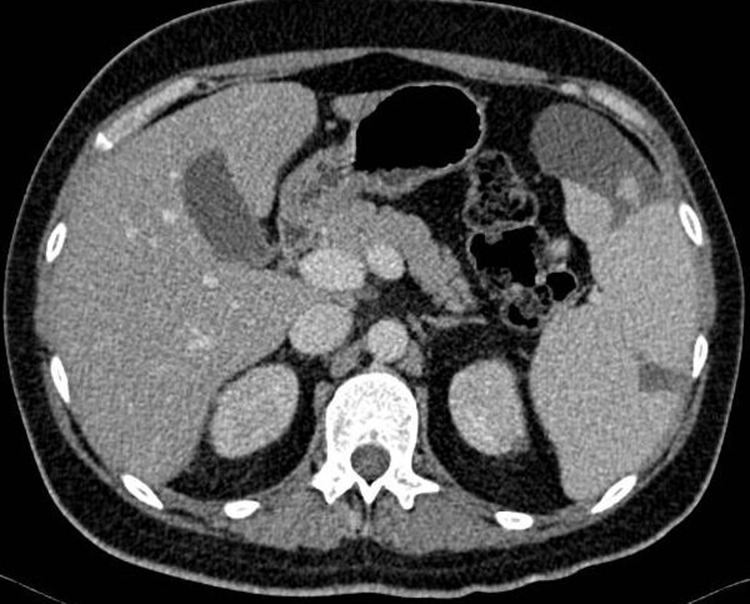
abdominal CT image showing multiple infarcts of the spleen
